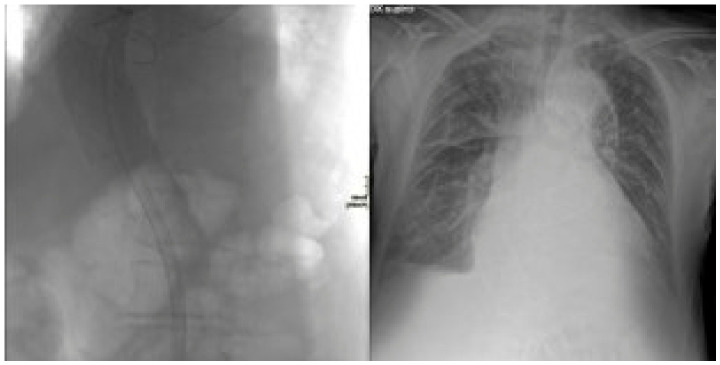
(Left): Digital subtraction angiography (DSA) of the thoracic aorta demonstrating endoleak within the aortic arch and descending aorta. (Right): Follow-up chest radiograph showing correct deployment and apposition of the endovascular stent. These findings highlight the complementary role of angiography and radiography in procedural guidance and post-treatment assessment.
